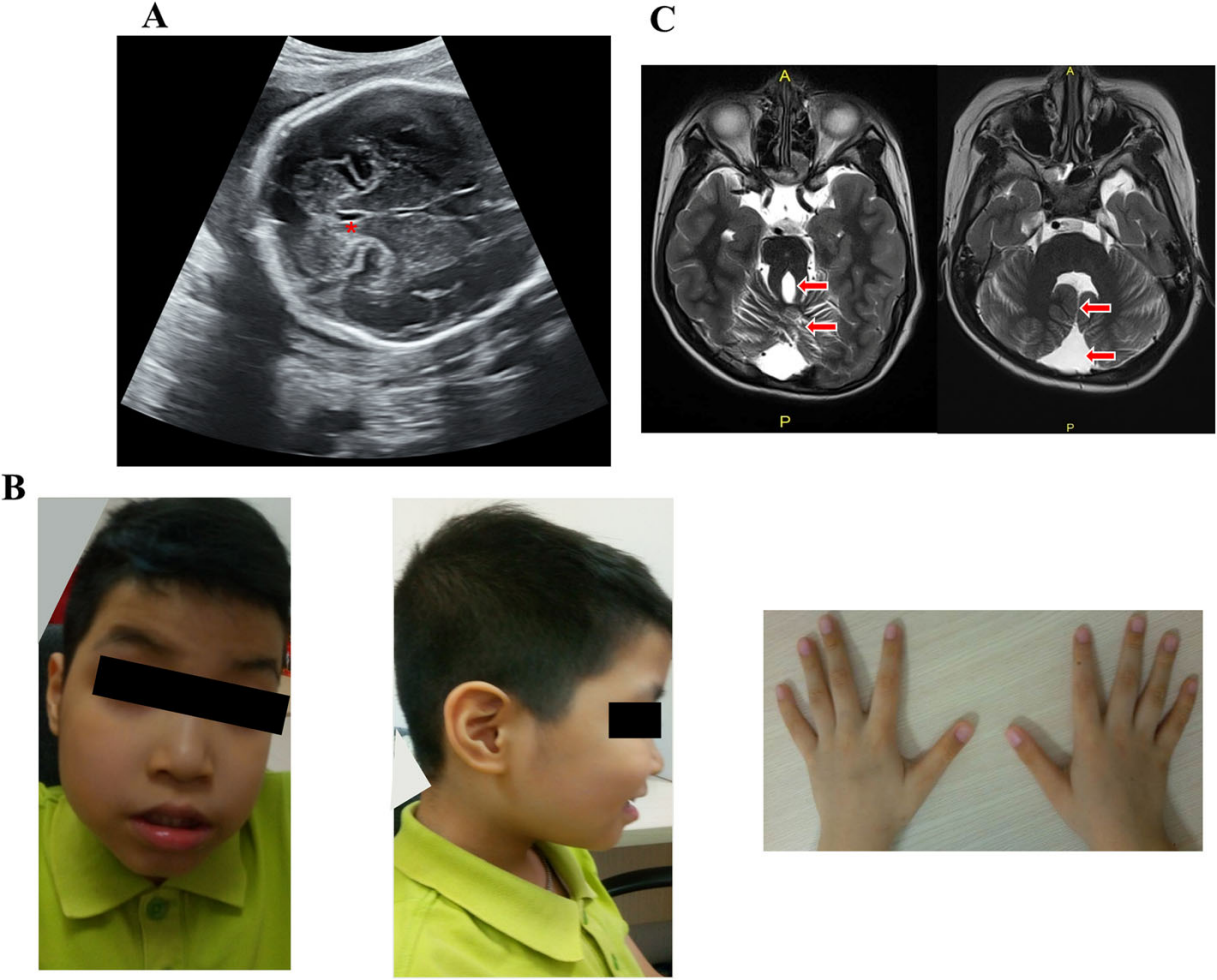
a Ultrasound at 26 + 6 weeks of gestation showing an abnormal enlarged fourth ventricle (red asterisk) and the ventricle floor is abnormal. b Photographs of the face (left image) and right profile (middle image) showing typical facial features of Joubert syndrome including prominent forehead, high arched eyebrows, bilateral ptosis, hypertelorism, lower lip eversion (left and middle image), mild clinodactyly of the fifth finger and tapered fingers (right image). c Axial MRI through the cerebellum and brain stem showing cerebellar vermis hypoplasia, thick and elongated superior cerebellar peduncles (red arrows, left image) and absence of the cerebellar vermis, deep interpeduncular fossa and the fourth ventricle has a bat-wing configuration (red arrows, right image)
